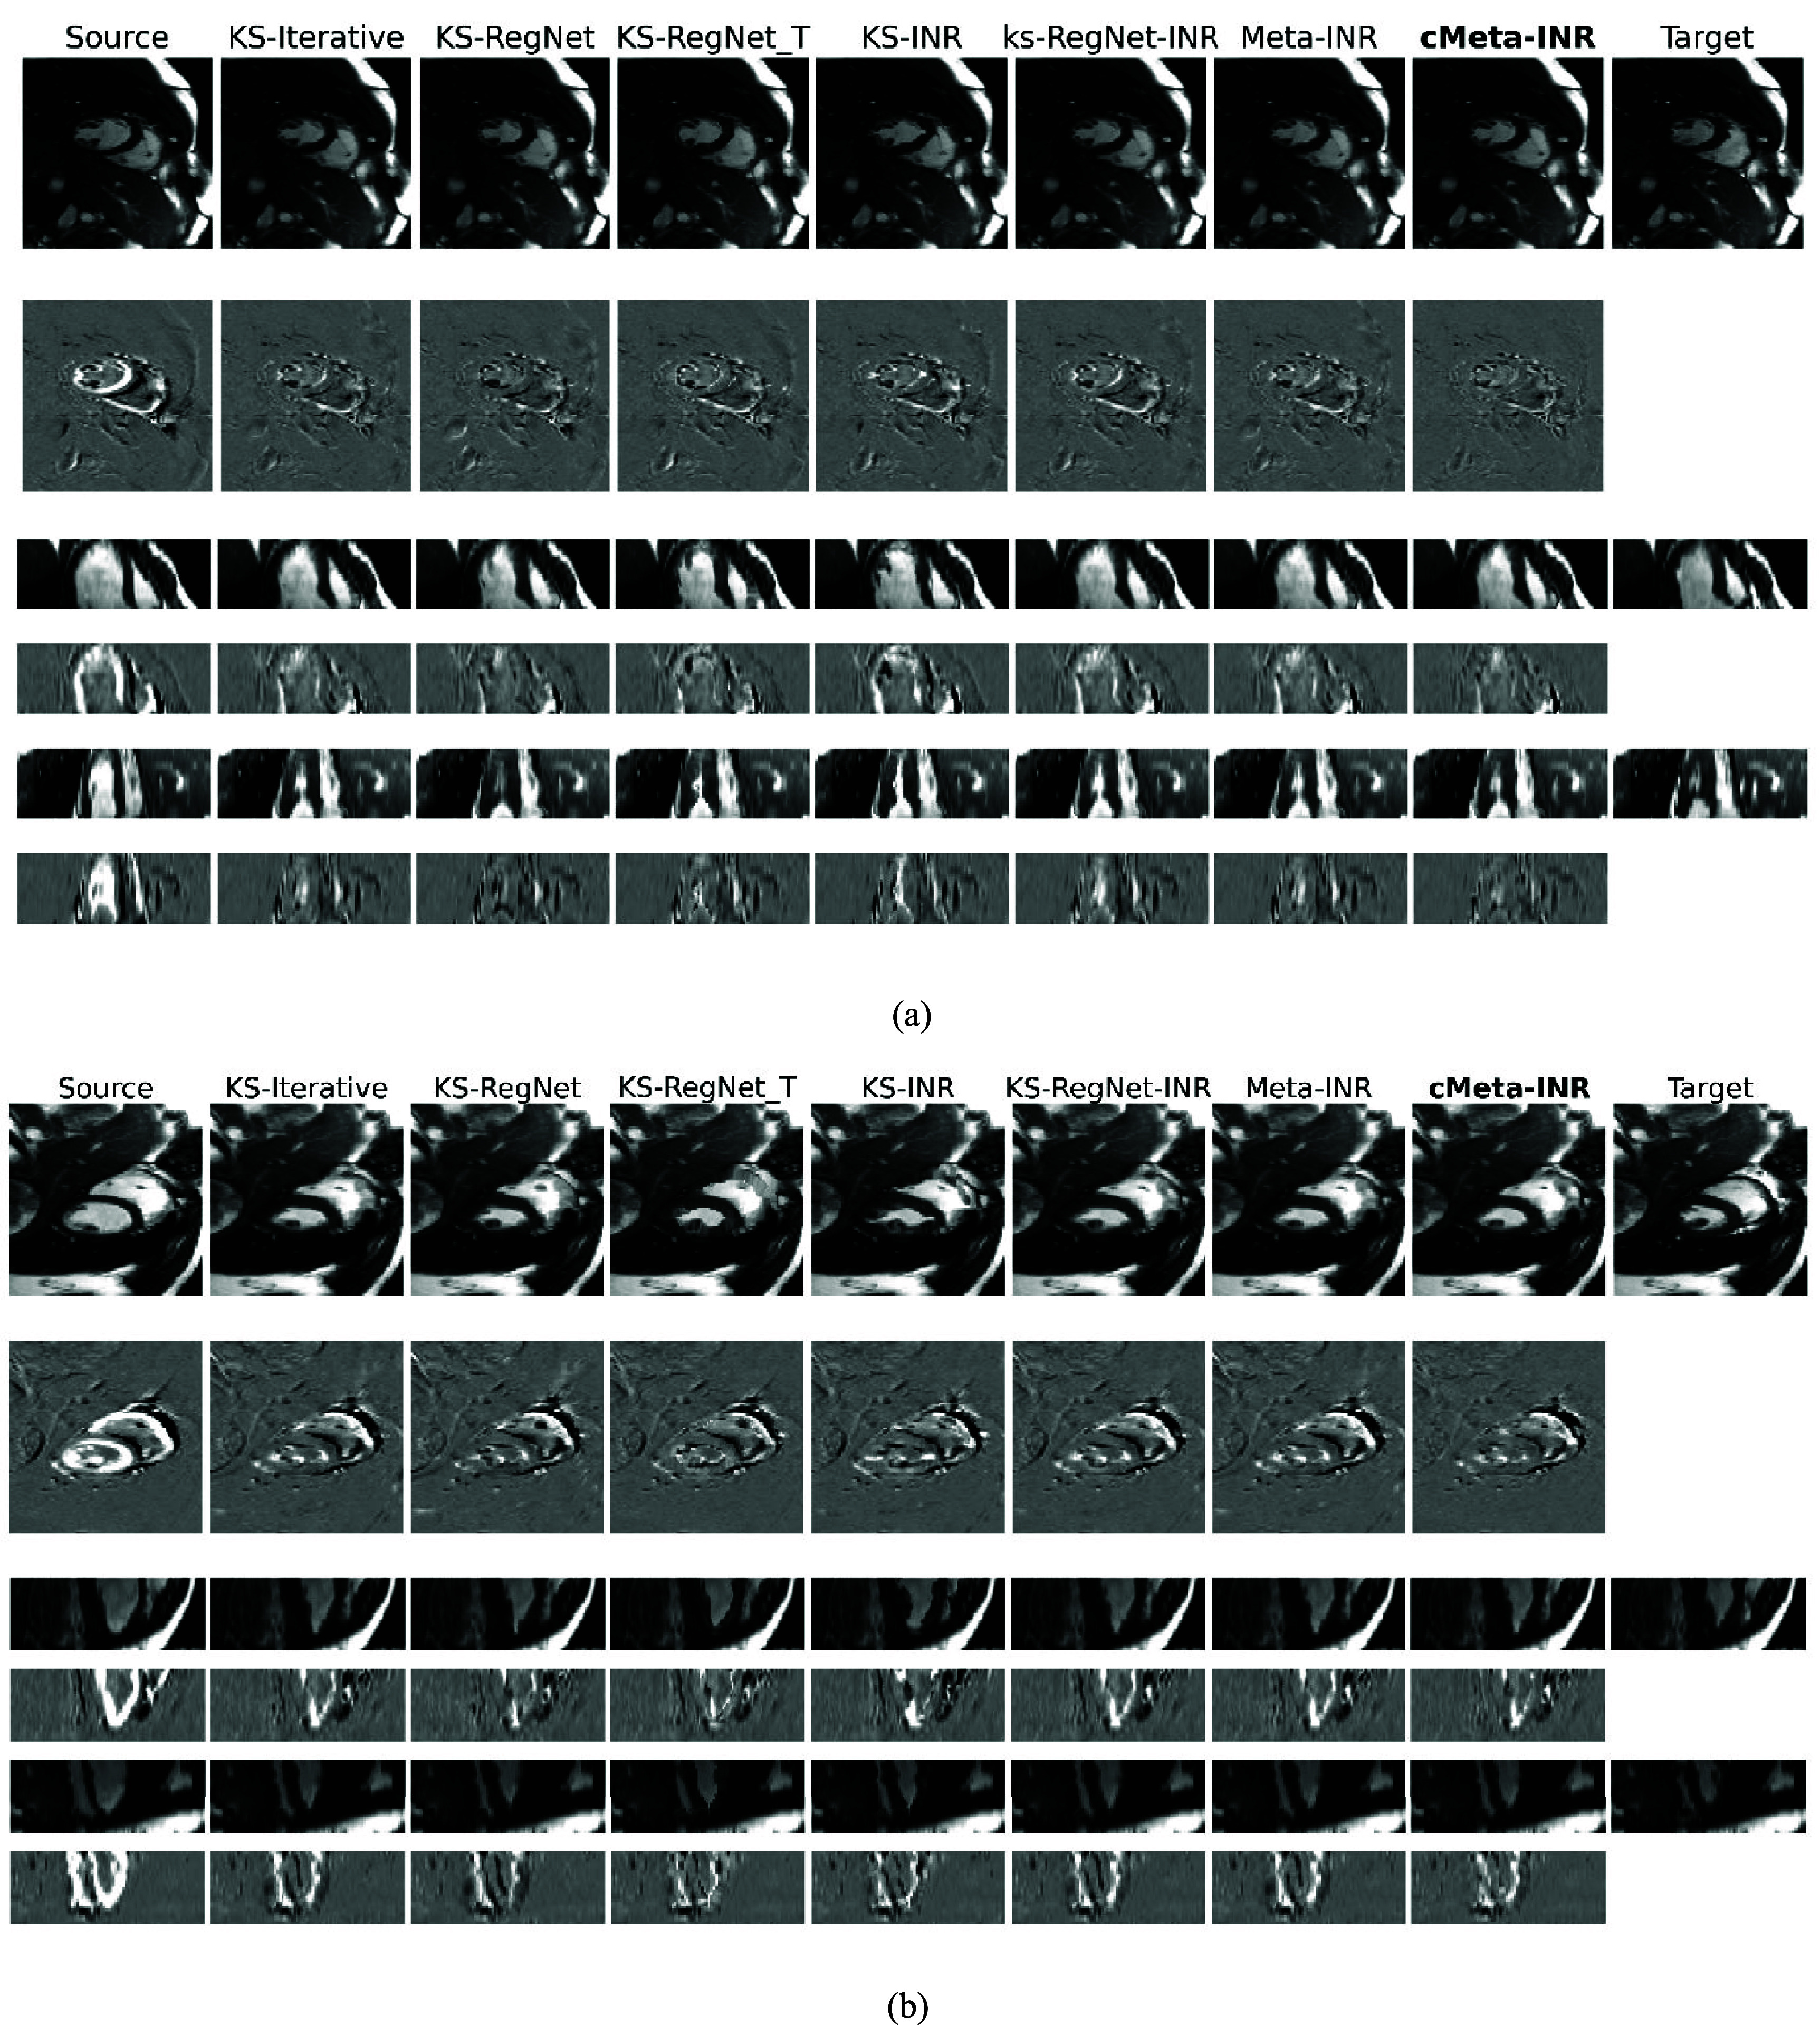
Comparison of cardiac MR images by different methods. Subfigures (a) and (b) present the images from different subjects in the axial, coronal, and sagittal views (odd rows), and the differences between these images and the target images (even rows). The spoke number used for *k*-space sampling is 13.
